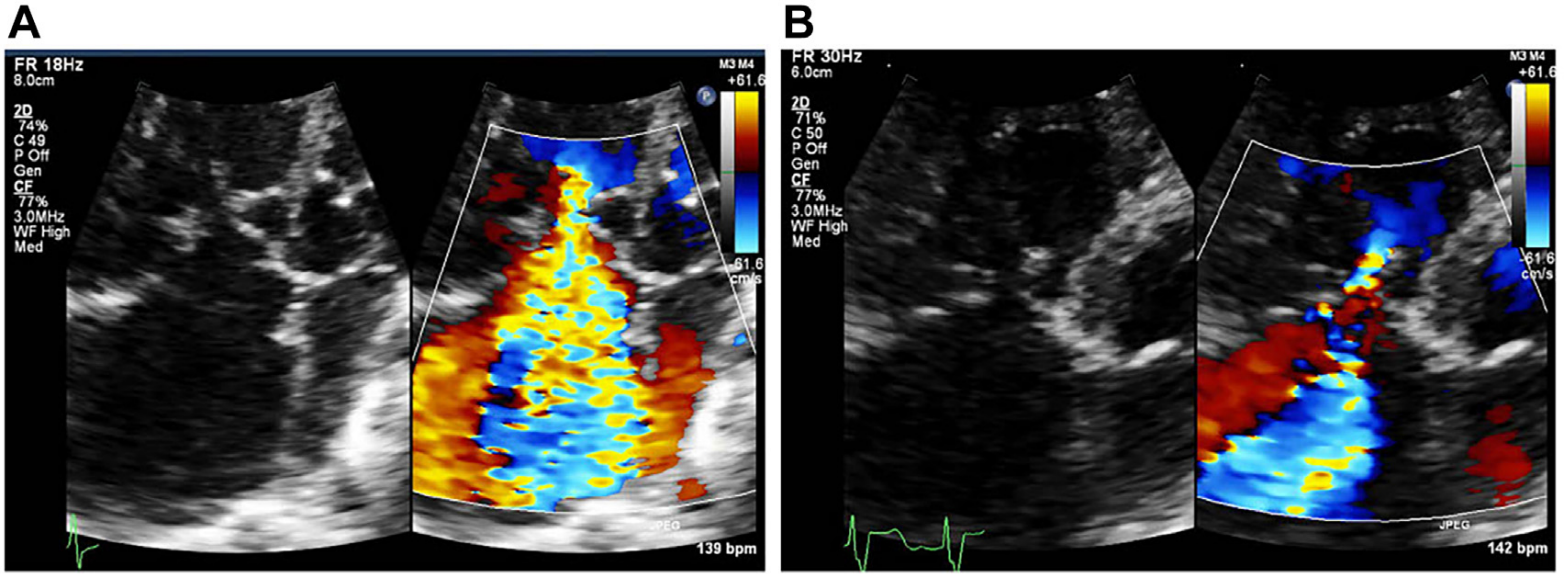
Transthoracic echocardiography revealed tricuspid regurgitation (TR) showing the 4-chamber view before and after surgery. (A) Massive TR due to the restricted movement of the shortened chordae attached to the septal leaflet. (B) Mild TR after the improved coaptation of the septal leaflet.
